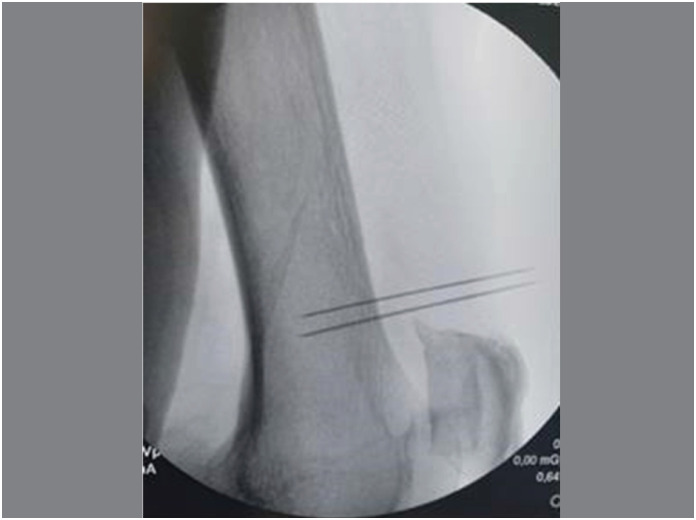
Positioning in Profile radiography for thermoregulation of the superior medial genicular nerve and superior lateral genicular nerve. rofile x-ray incidence of medial and lateral superior genicular nerves radiofrequency.
